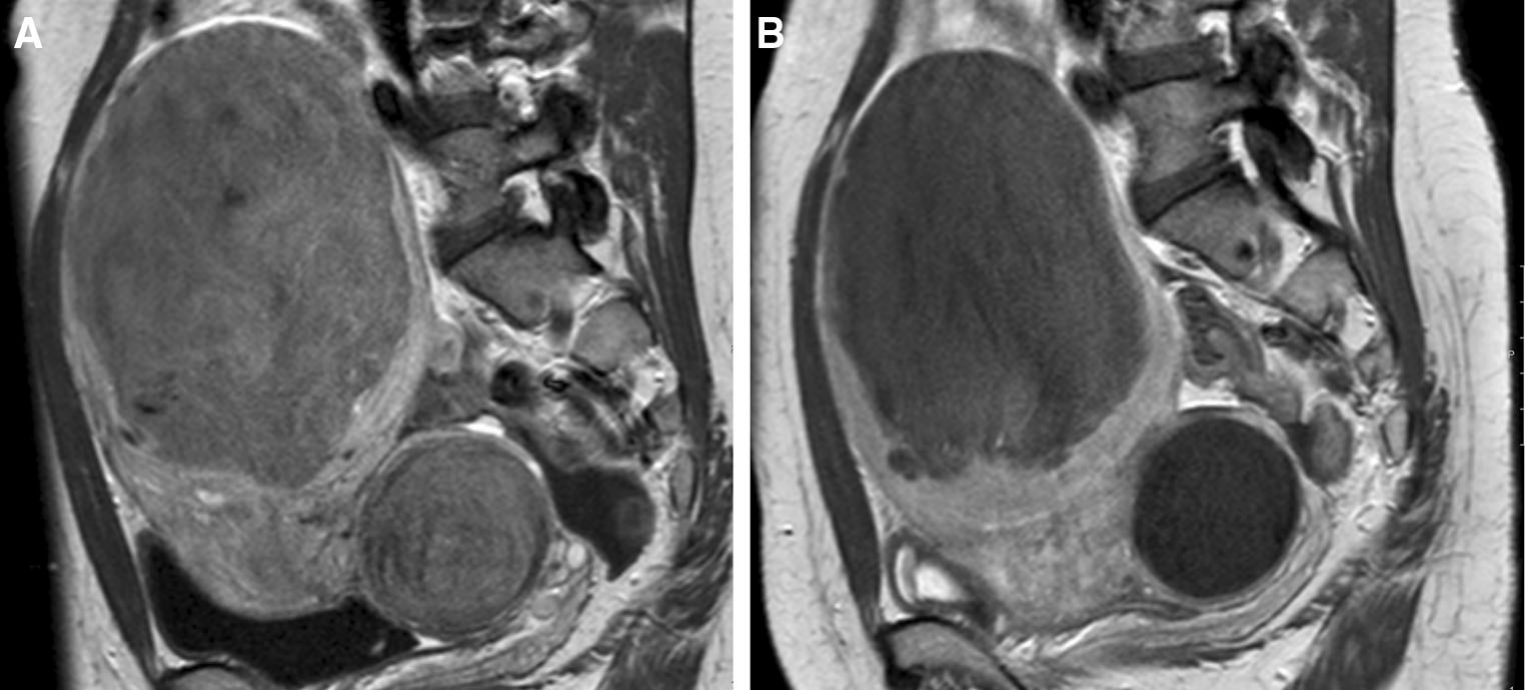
MRI (*SAG T1* − *TSE* − *HR contrast* +) imaging of cervical leiomyomatas in combination with uterine body fibroids. *Note* Patient no. 6, MR images (sagittal T1 − TSE − HR + contrast) of leiomyomata in the uterine body and cervix. A Both the cervical leiomyomata (106 cm^3^) and the uterine body leiomyomata demonstrated full enhancement prior to UAE. B Follow-up imaging 3 months after UAE with 5% enhancement of the leiomyomata in the uterine body and no enhancement of the cervical leiomyomata, as a result of complete infarction after bilateral UAE (2cc700 µm, 4cc900 µm and 4cc1200 µm microspheres, Embozene™). The cervical leiomyomata volume 3 months after UAE was 77 cm^3^ with a volume reduction of 27%
